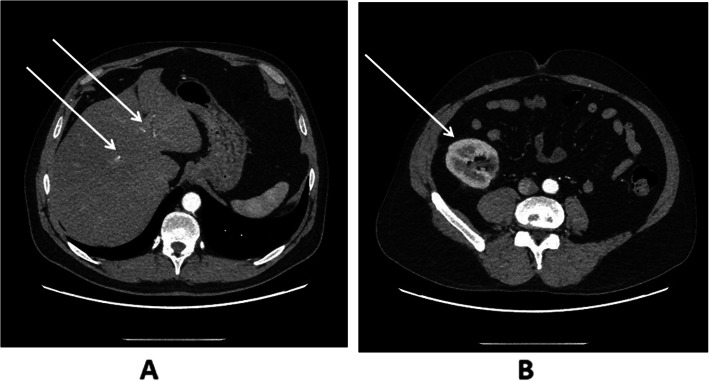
Computed tomography (CT) of the abdomen. Liver steatosis (white arrows) (Fig. 2 A). Transplanted kidney in the right iliac fossa (white arrow), 116 × 62 mm size, without abnormalities (Fig. 2 B)
